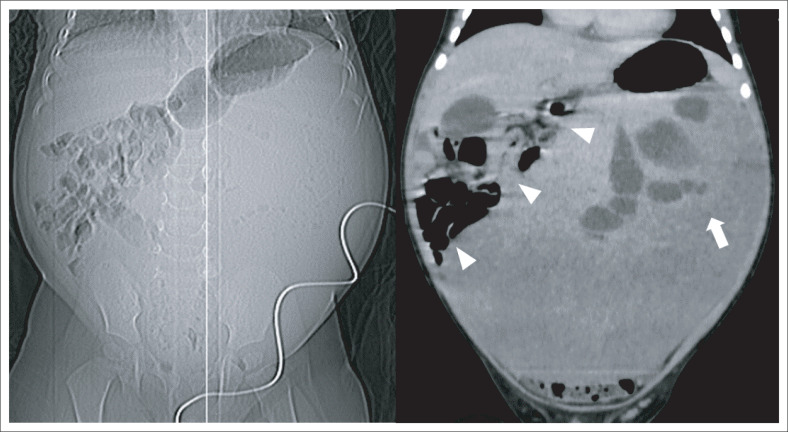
Follow-up imaging – Abdominal computed tomography scout image and coronal contrast-enhanced imaging (portal venous phase) demonstrates the mass to have shifted into the left abdomen (arrow), causing displacement of the bowel to the right (arrowheads). This reveals the mass to be mobile and separable from the right and left liver lobes.
